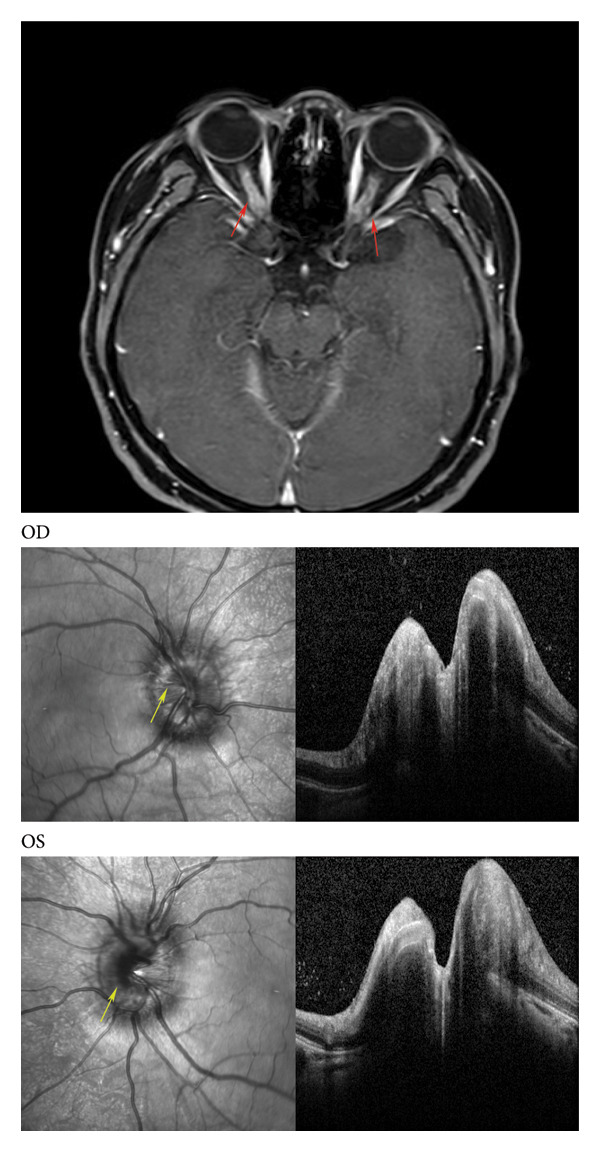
Magnetic resonance imaging and optical coherence tomography of patients with optic neuritis after COVID‐19 infection. A 23‐year‐old female patient complained of right eye pain and decreased vision after infection with COVID‐19. Her vision was 0.08 and 0.5 in the right and left eyes, respectively. Magnetic resonance imaging in T1‐weighted imaging fat‐sat + C sag showed that the optic nerve margins were significantly strengthened (red arrow). OCT showed swollen optic discs in both eyes with blurred borders (yellow arrow). (OD: right eye, OS: left eye).
